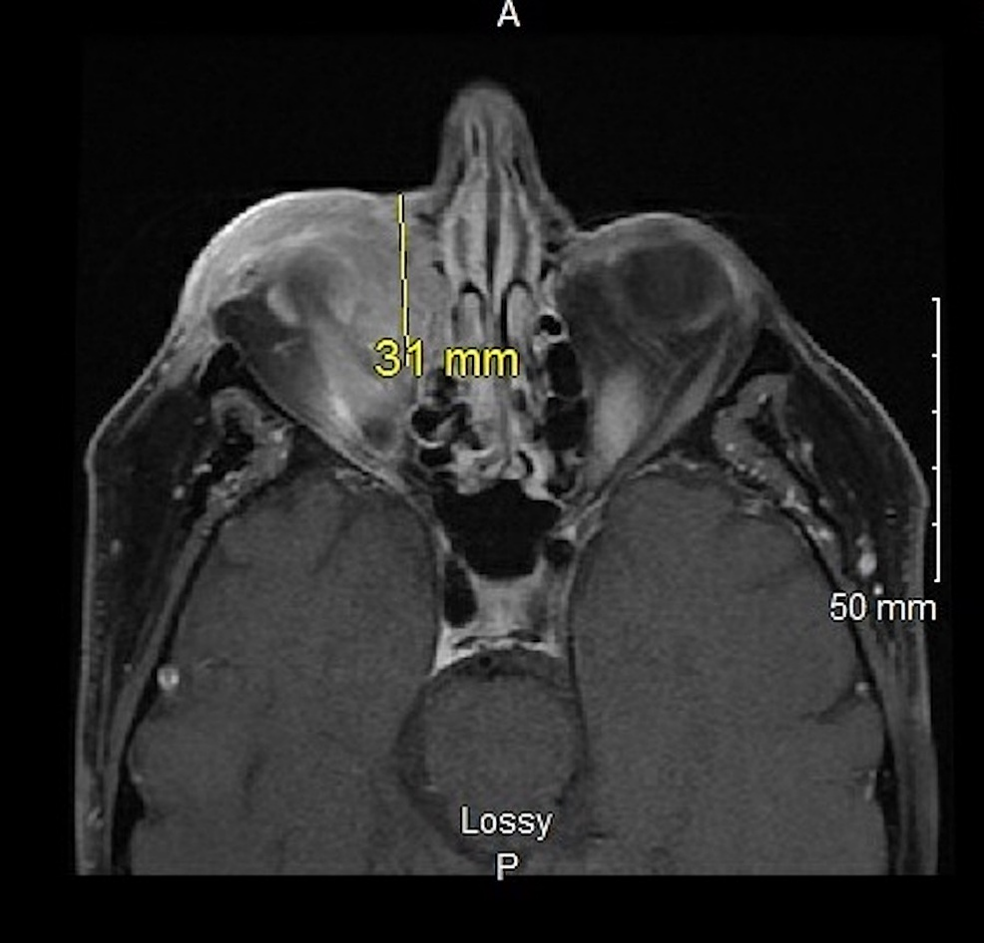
Cranial MRI axial section showing the infiltrating right orbit tumor. MRI: magnetic resonance imaging
